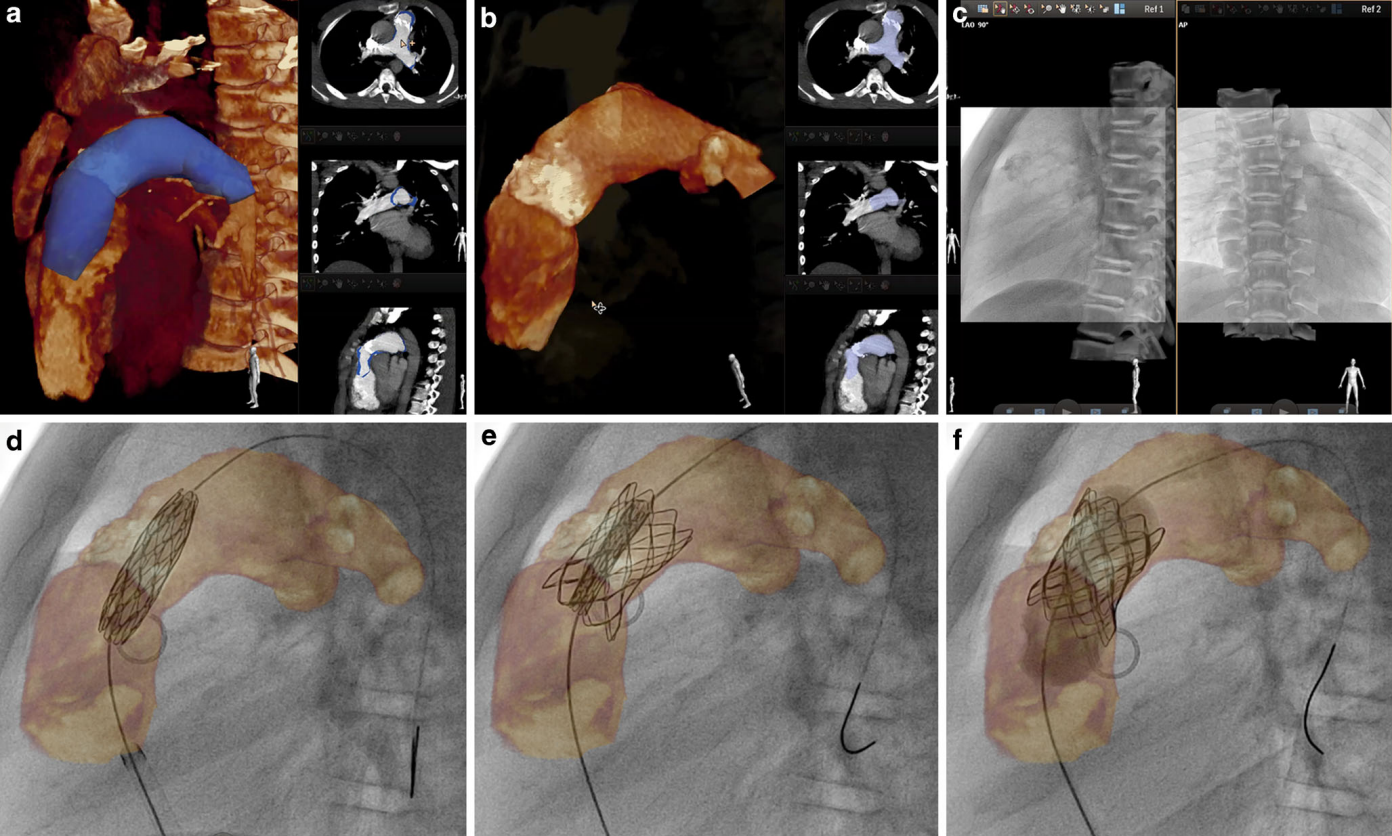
VesselNavigator assisted percutaneous pulmonary valve implantation. Automatic three-dimensional (3D) reconstruction (a, *left panel*) and row scans (*right panels*) from pre-registered computed tomography were manipulated to outline the conduit and the proximal pulmonary arteries (b). In addition to bony structures, heavy conduit calcifications were utilised to enhance manual 3D image fusion with live fluoroscopy (c). The 3D roadmap was utilised to guide creation of the landing zone (d) and subsequent implantation (e, f) of a Melody valve (Medtronic Inc.)
